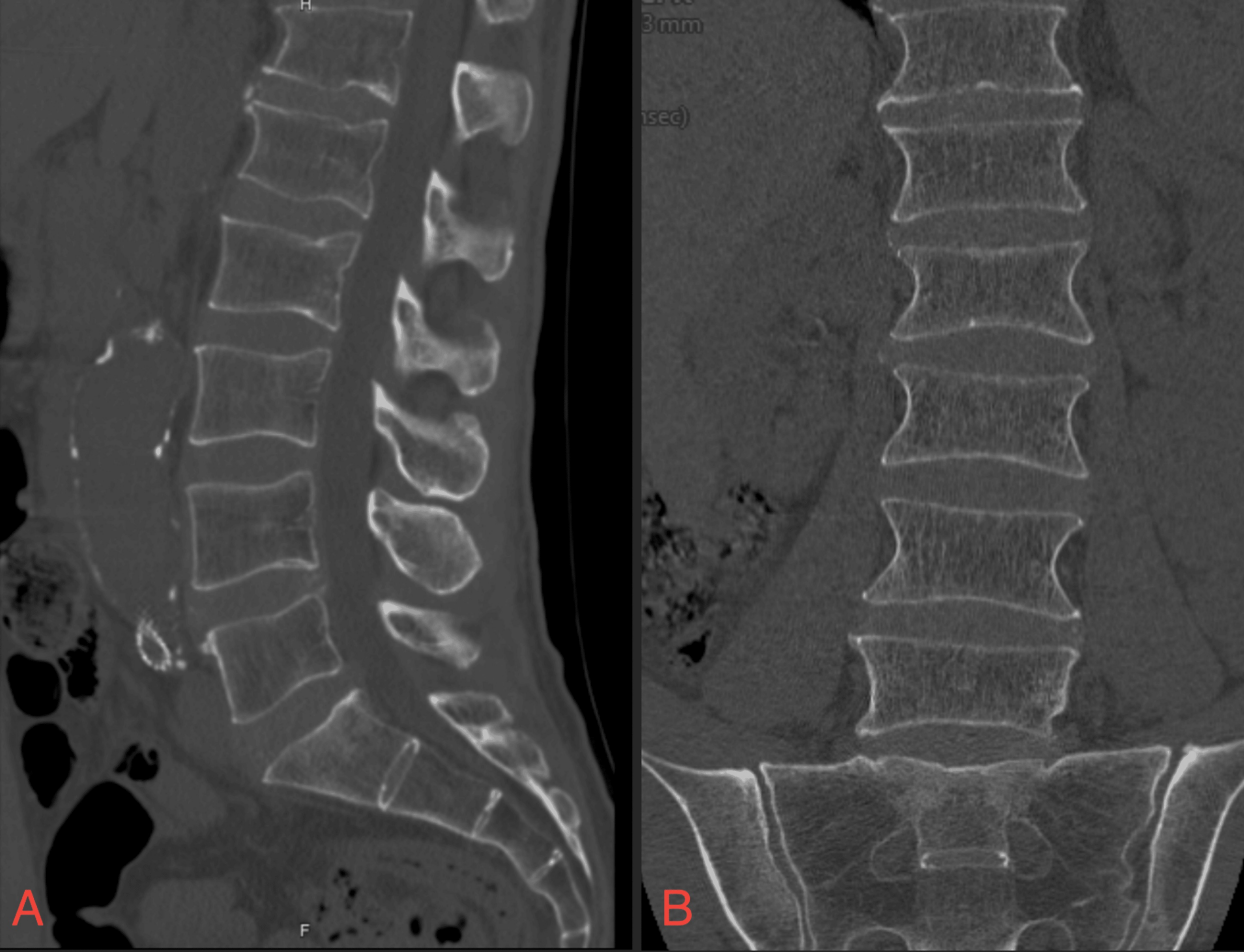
Sagittal (A) and coronal (B) images from CT scan of lumbar spine
